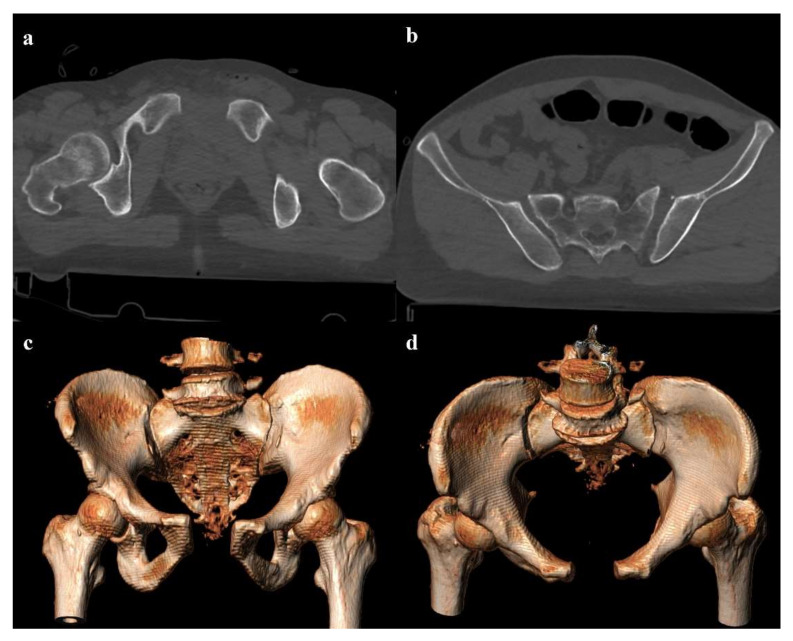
Anterior–posterior compression fracture, type 3. Axial CT images (a,b) and three-dimensional volume-rendering CT reconstructions in AP and inlet views (c,d) show a pubic symphysis diastasis of 4.2 cm, anterior and posterior left side sacroiliac joint diastasis, and anterior widening of the right sacroiliac joint.
